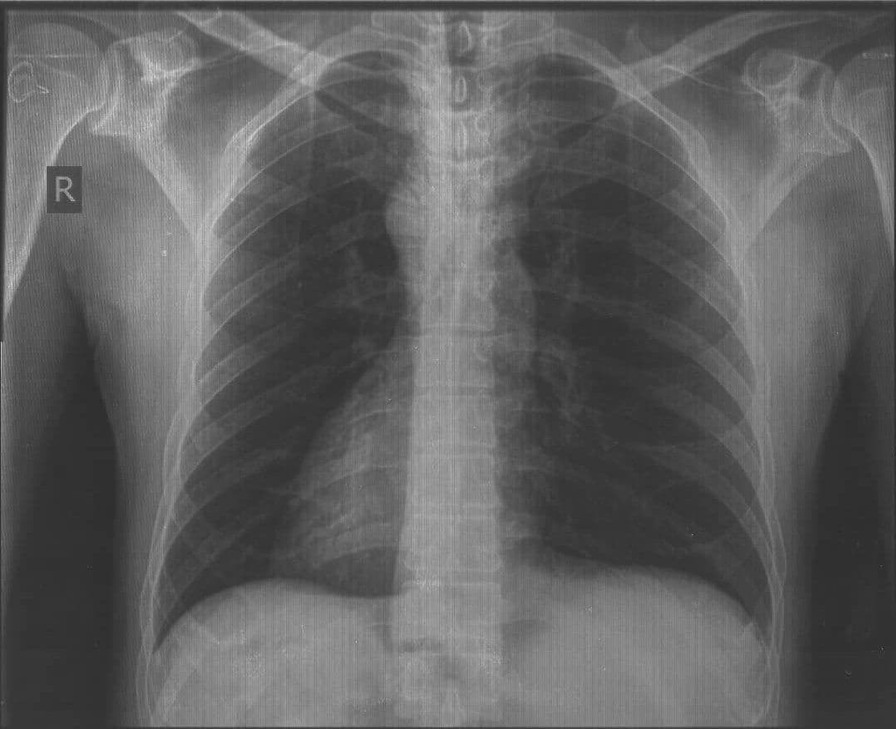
Chest X-ray showing dextrocardia
